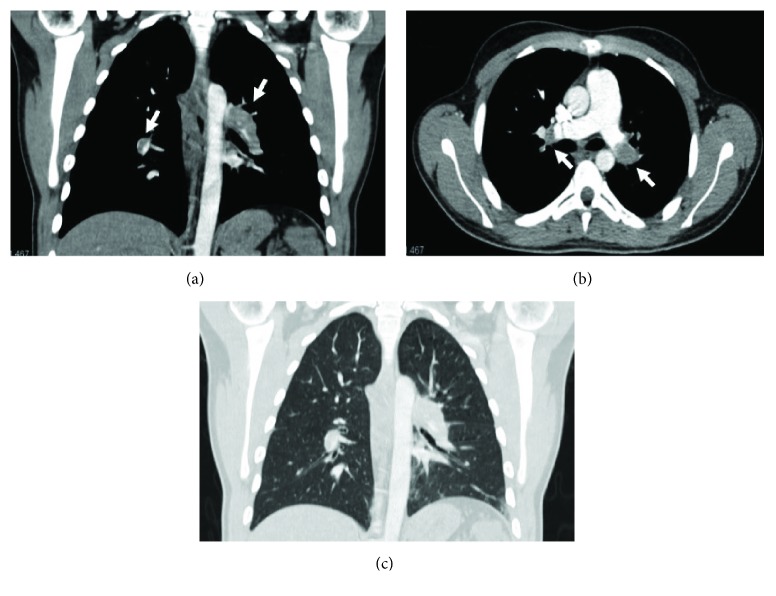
An 18-year-old male with Crohn's disease in the acute phase and no additional comorbidities, who presented with mild shortness of breath. Coronal and axial reconstruction of contrast-enhanced chest CT reveals bilateral pulmonary embolism in both main pulmonary arteries (arrows, a-b). Coronal reconstruction for lung parenchyma (c).
